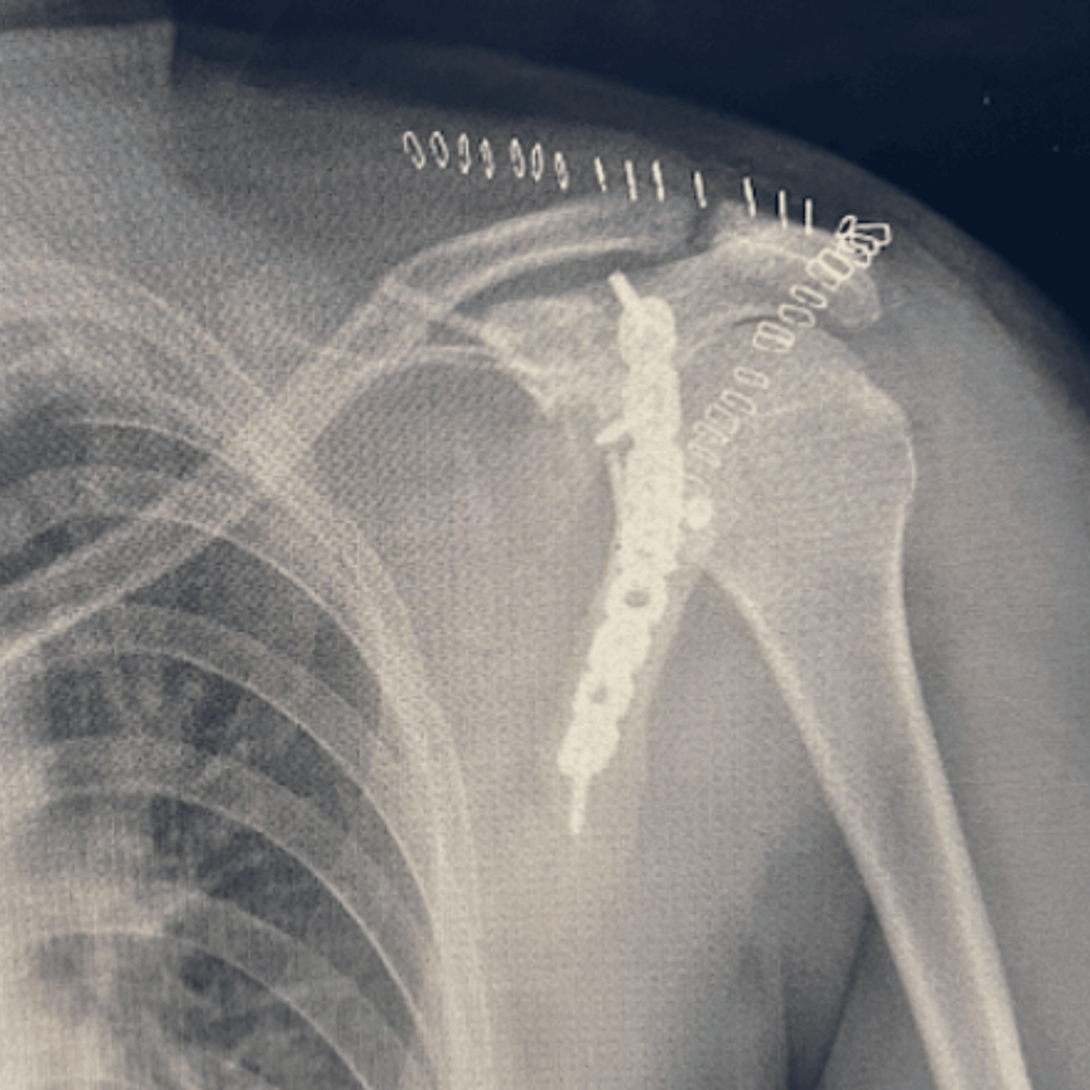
Postoperative AP radiographic view of the left shoulder.
